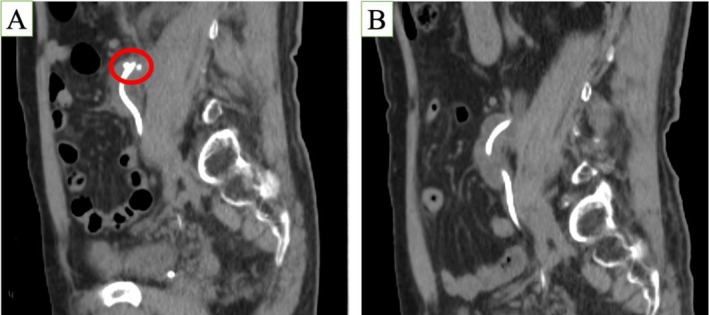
(A) A ureteral stone was found in the upper side of the anastomosis. (B) Computed tomography on postoperative Day 181 showed no stones.
